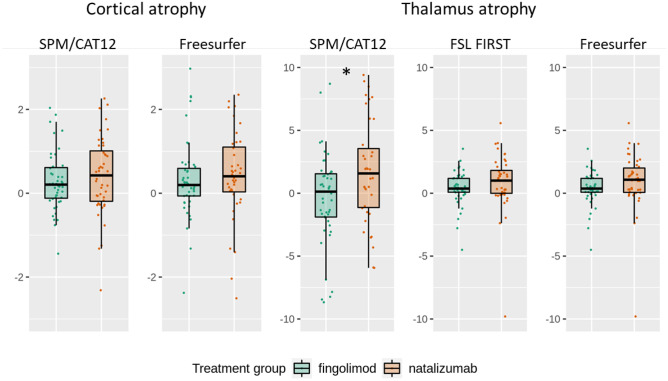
Comparison of longitudinal MRI measures of grey matter atrophy subordinate to brain atrophy. Cortical and thalamic atrophy values (annualized percentage changes of cortical thickness and thalamus volumes). Asterisks indicate significance at *p* < 0.05
